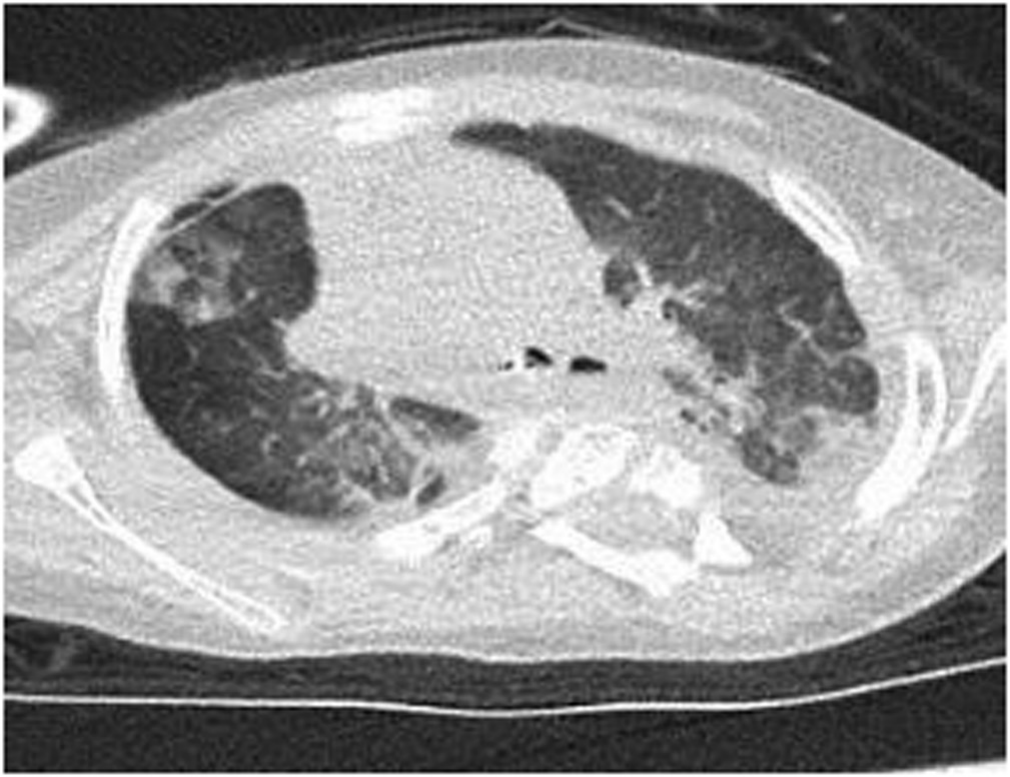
High-resolution computed tomography (HRCT) of a 3-year-old girl with VACTERL association, epilepsy, cognitive/motor delay, and COVID-19 pneumonia: Areas of complete/incomplete consolidation with sporadic air bronchograms in bilateral lung parenchyma and ground-glass opacities in the bilateral lung parenchyma. COVID-19: coronavirus disease 2019.
